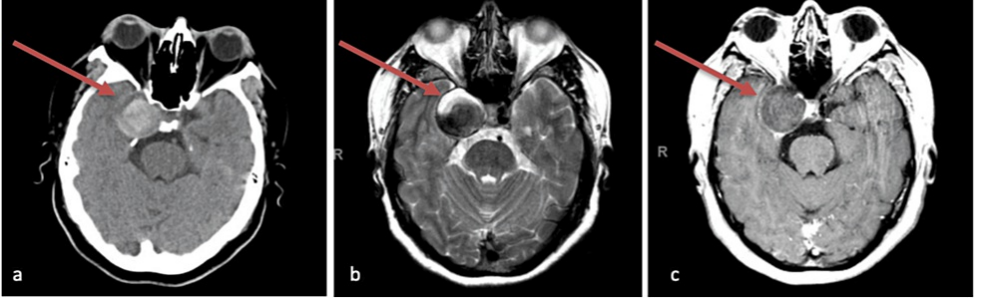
Brain CT and MRI Figure 1a shows a brain CT without contrast, demonstrating the presence of a 3.2 cm dilatation of the right internal carotid artery, indicating a thrombosed aneurysm. Figure 1b is an MRI with and without contrast. Figure 1c confirms the presence of the aneurysm.
